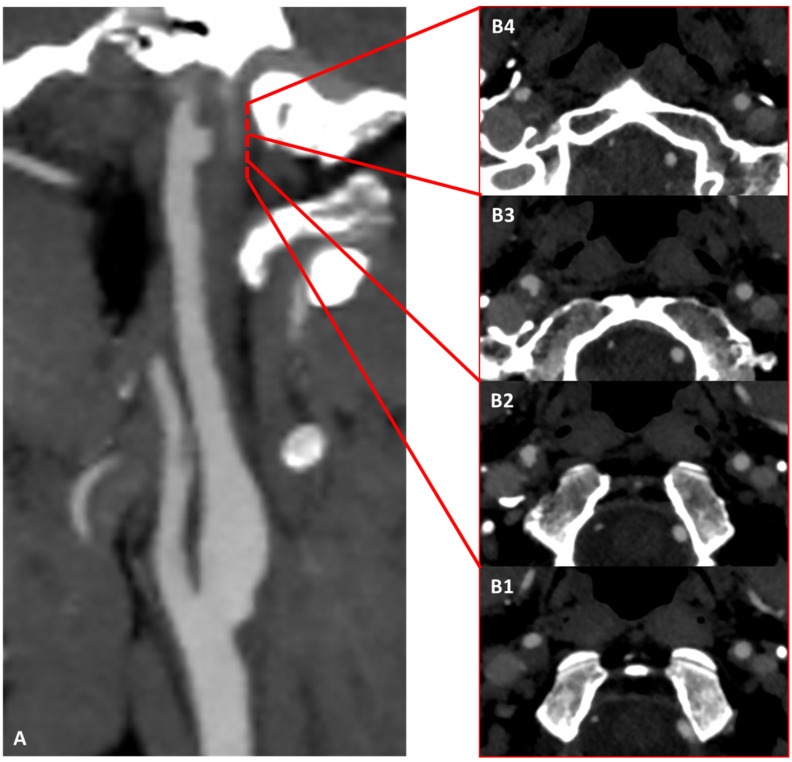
CTA in MIP/MPR reconstruction of the right ICA in oblique plane (A), showing an atheroma with ulceration on the posterior wall of the prepetrous segment. (B1–B4) are the corresponding source axial slices in a proximal-to-distal sequence, at a level corresponding to the intersection points of the continuous red line on the dotted red line in panel (A).
